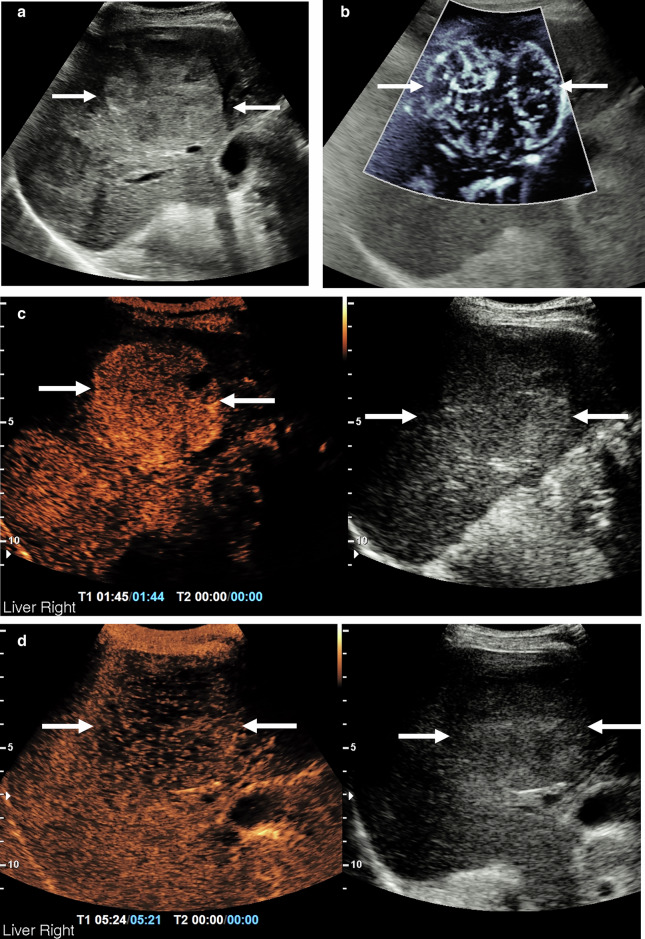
Surveillance ultrasound in a patient with known hepatitis B viraemia lost to follow-up; hepatocellular carcinoma. a Multiple focal liver lesions seen in the right lobe of the liver, the largest measuring 58 × 55 mm (arrows), of mixed reflectivity, suggestive of hepatocellular carcinoma. b Microvascular imaging demonstrating markedly disordered internal vascularity of the focal liver lesion (arrows). c The CEUS examination depicts rapid early arterial enhancement and sustained enhancement at 1 min 44 s. d The delayed portal venous phase depicts washout at 5 min 21s, indicating a CEUS-LIRADS 5 lesion
